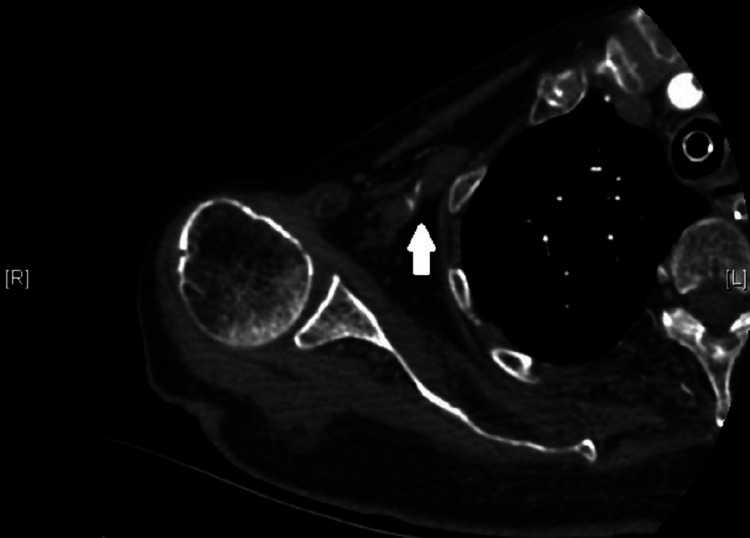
Computed tomography scan showing arterial thrombosis
